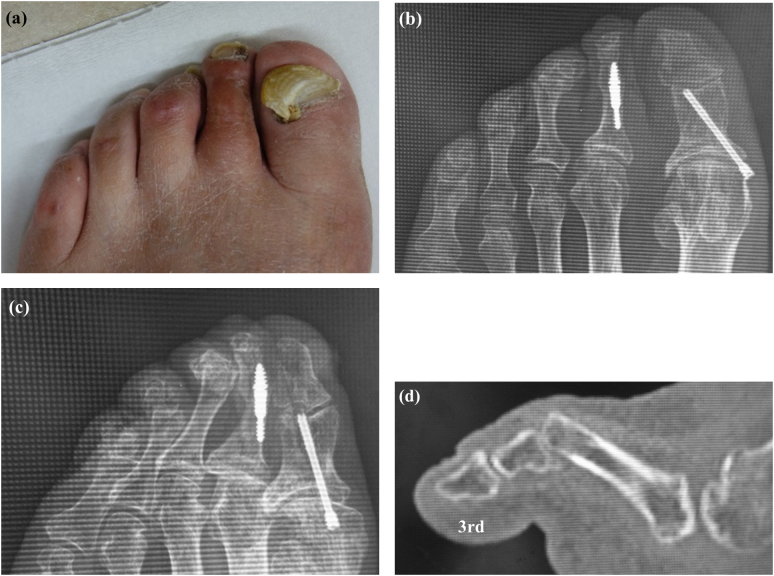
Clinical photograph showing callus formation over the third and fourth proximal interphalangeal joints (a). Radiographs showing third and fourth hammer toe deformity on anteroposterior (b) and oblique (c) views. Sagittal computed tomography image showing hyperflexion of the third proximal interphalangeal joint (d).
